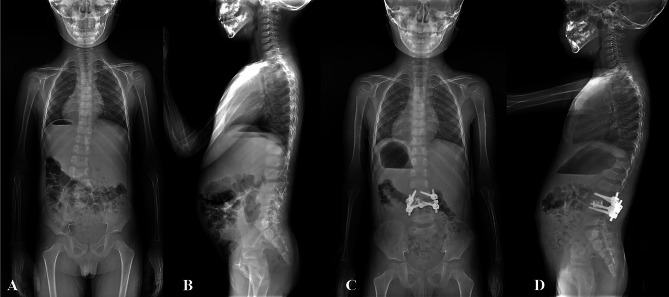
A case of partially segmented hemivertebra with concomitant cardiac abnormalities A 5/M, diagnosed with congenital scoliosis, he had a partially segmented hemivertebra at L3 segment (a, b). The ultrasonic cardiography of him showed mitral valve redundancy. And he underwent posterior hemivertebra resection and short segment fusion surgery, the X-ray showed L3 hemivertebra was totally resected (c, d)
